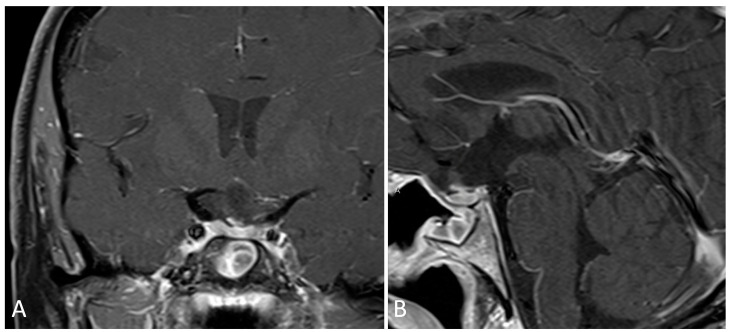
Postoperative imaging consistent with gross total resection of craniopharyngioma. (A) Coronal T1 post-contrast MRI. (B) Sagittal T1 post-contrast MRI.
